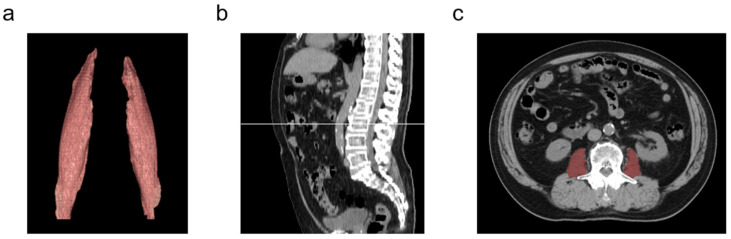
Evaluation of the psoas muscle area in a representative patient. (a) Image of the psoas muscle isolated using an automatic analysis program on the Ziostation2 workstation. (b) Sagittal computed tomography (CT) image; the white line indicates the mid-level of the third lumbar vertebra (L3). (c) Axial CT image with the psoas muscle highlighted in red.
